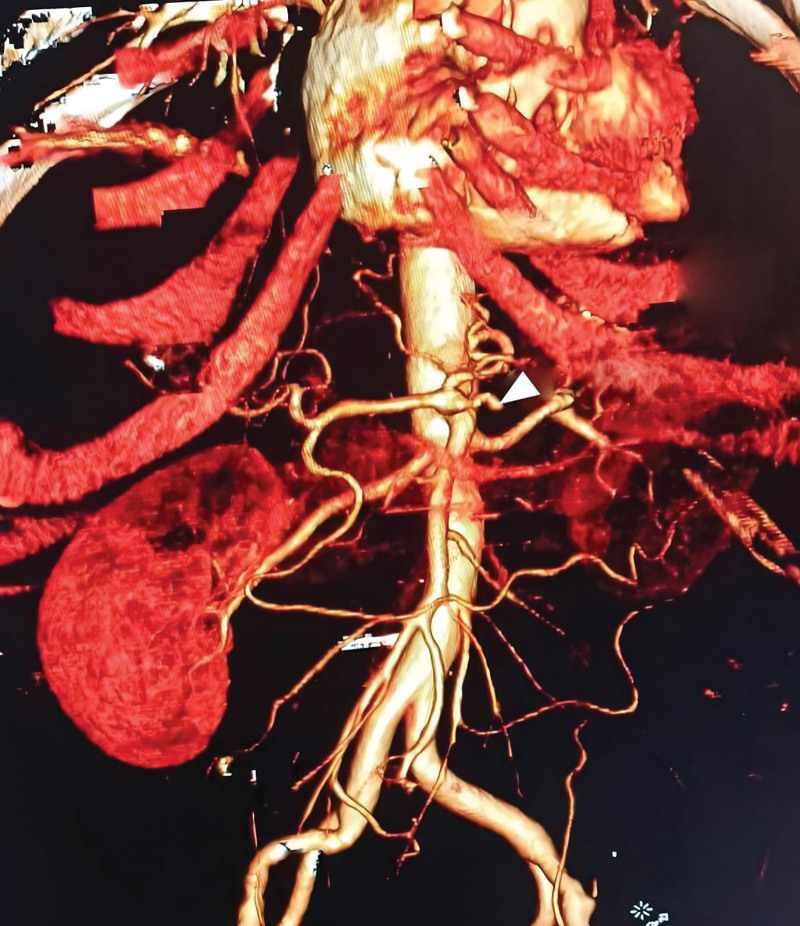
Follow-up three-dimensional reconstruction MSCT demonstrates the splenic artery stump (white arrow) with the absence of any other visceral aneurysm. MSCT = multi-slice computed tomography.
